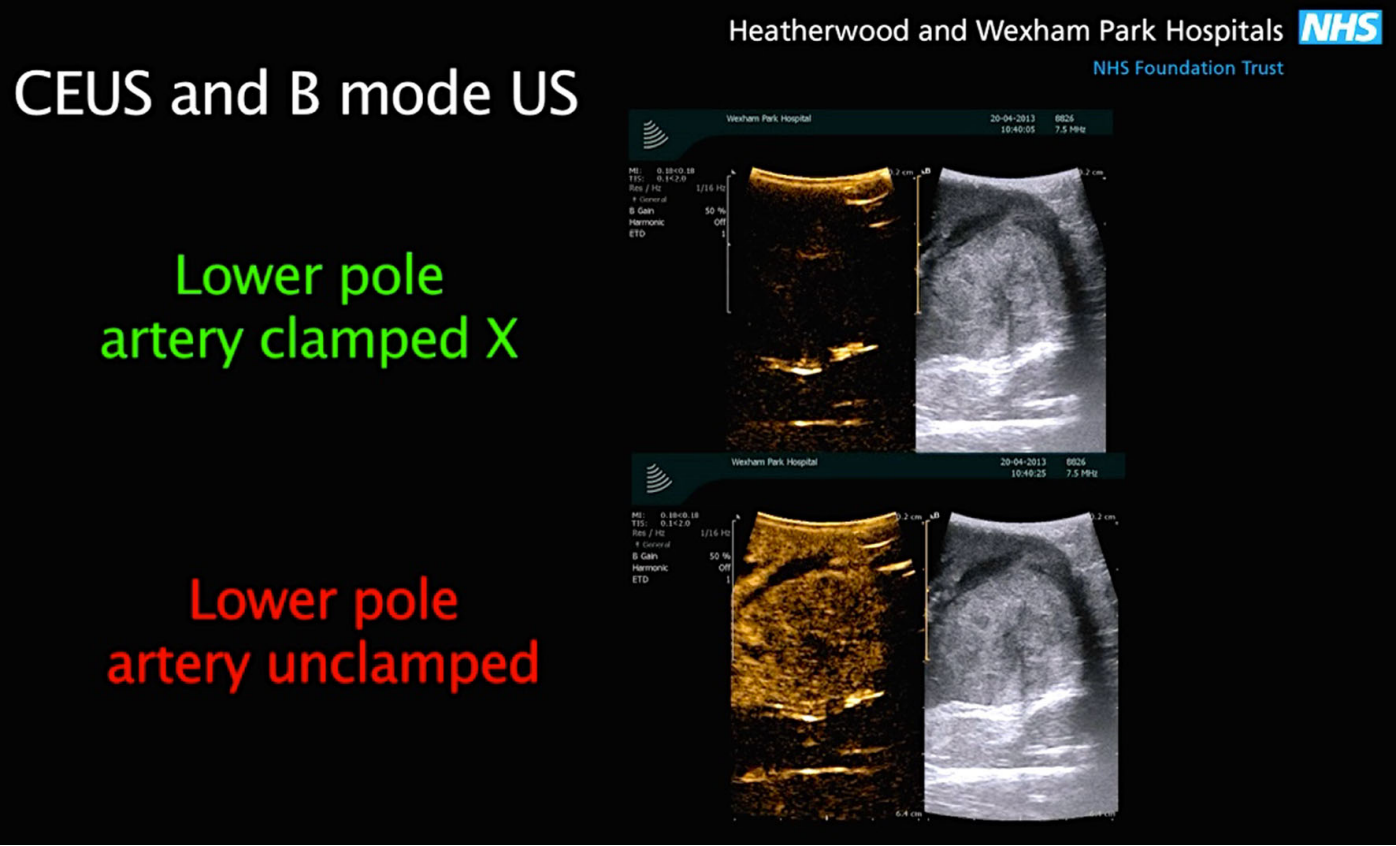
the difference between a conventional B-mode images on the right and the contrast-enhanced mode images on the left. SonoVue microbubble contrast agent was injected intravenously. The contrast-enhanced ultrasound mode in the monitor shows the circulation in the kidney and the segment containing the tumor in the lower pole. This facilitates and confirms selective ischemia to the desired renal segment
